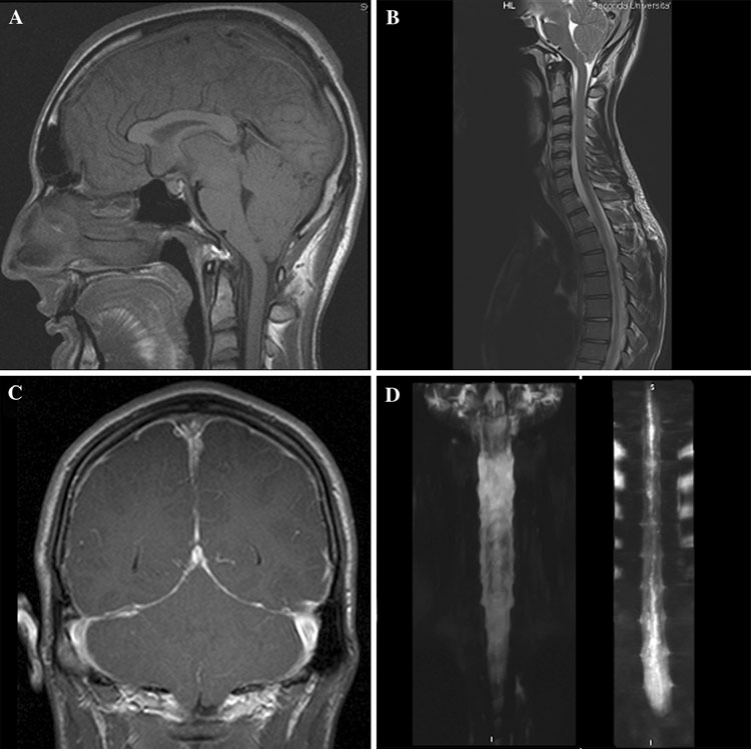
a Sagittal T1-weighted brain MRI, b sagittal T2-weighted spinal MRI, c coronal T1-weighted brain MRI post-gadolinium contrast administration, d MR myelogram of upper and lower spinal subdural space
